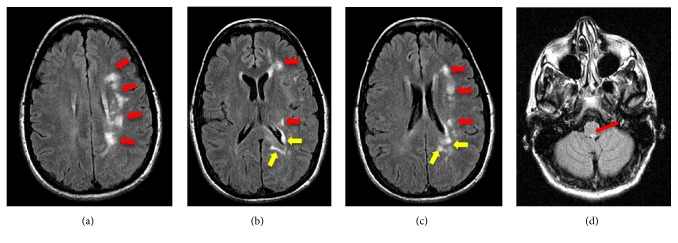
Axial brain MRI (a-d) depicts left-sided (a-c) cerebral white matter lesions (red arrows), periventricular lesions (b and c) of the left frontal and posterior horns of the lateral ventricles (yellow arrows), and T2-hyperintense foci of the midbrain (d) corresponding to the medial longitudinal fasciculus (red arrow).
